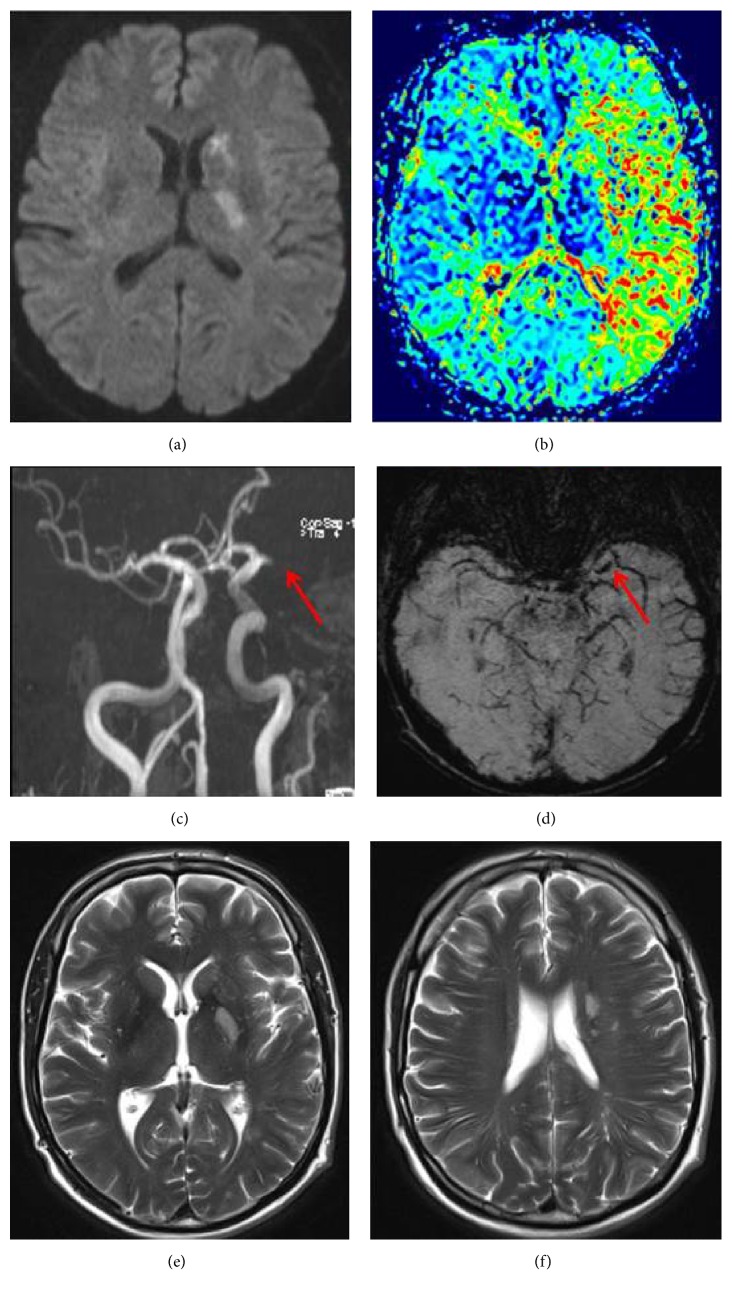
Cerebral magnetic resonance imaging 13 hours after stroke onset with a small diffusion lesion in the left basal ganglia and corona radiata with a complete perfusion deficit of the left MCA territory (a, b). Persistent occlusion of the MCA on magnetic resonance angiography ((c), red arrow) and susceptibility weighted imaging ((d), red arrow). T2 weighted imaging 24 hours after the intervention showing a small left sided basal ganglia infarction (e, f).
